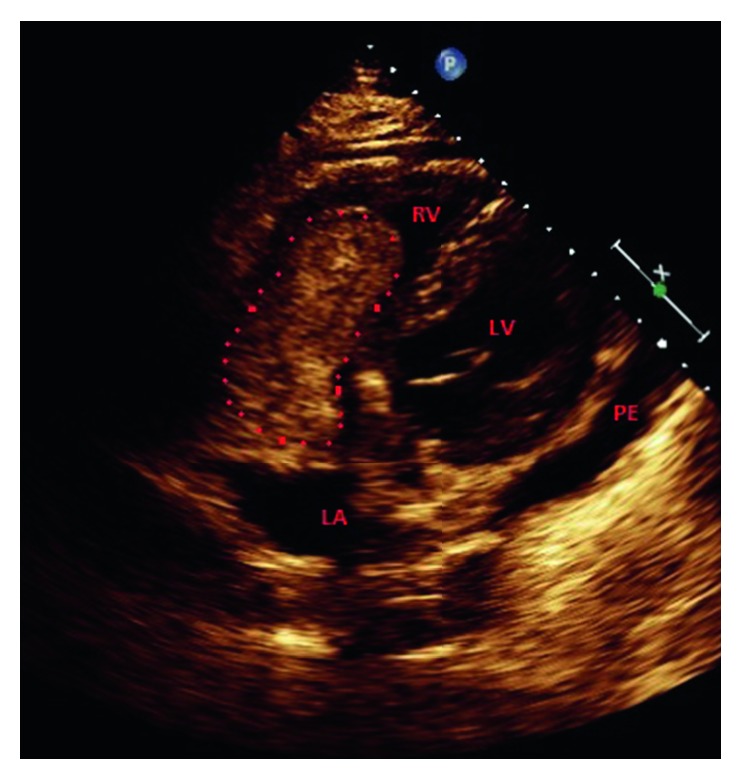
Transthoracic echocardiogram apical four chamber view showing the mobile mass (dotted line) within the right side of the heart. RV, right ventricle; LV, left ventricle; LA, left atrium; PE, pericardial effusion.
